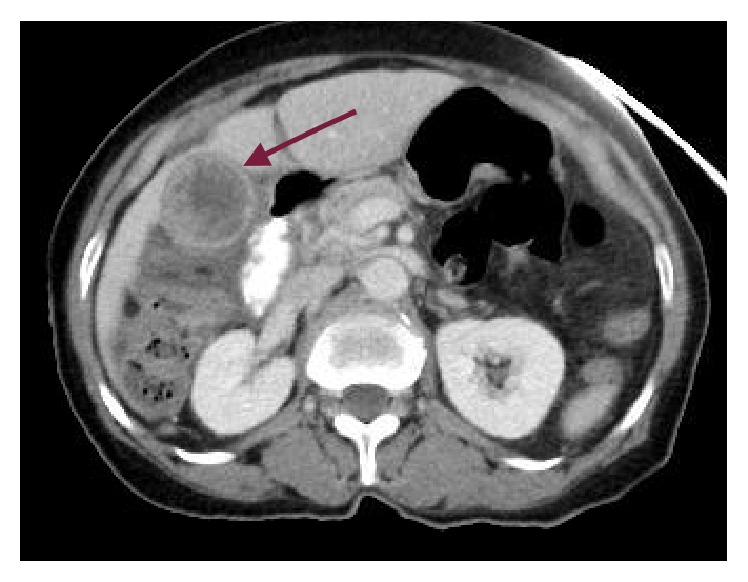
Abdominal CT showing irregular tissue mass in the gallbladder fundus (arrow).
